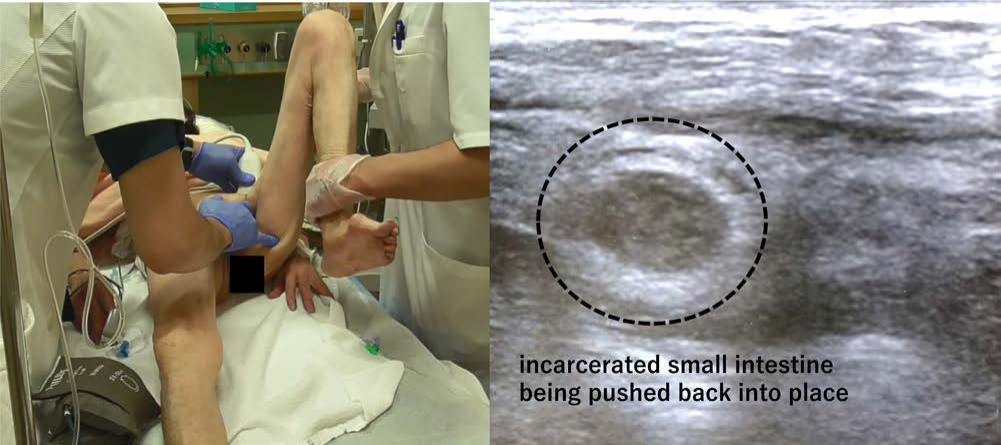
Step 1 and step 2. The surgeon places the transducer in the left hand on the groin area of the patient (just cranial to the inguinal ligament) while the right hand pushes the hernial sac. The assistant places the hip joint and the knee joint in a flexed position. Subsequently, the assistant slightly rotates the hip joint laterally, thus widely opening the hernial orifice. The ultrasound image shows the incarcerated small intestine being pushed back by the surgeon’s right hand.
